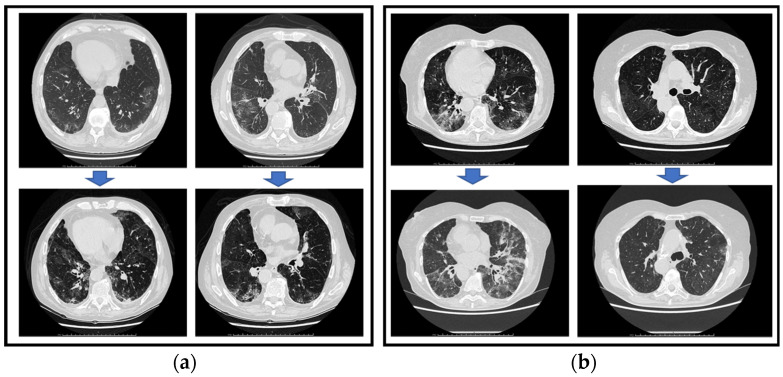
Chest CT scan before (upper row) and after (lower row) CP treatment. (a) Case 1; (b) Case 2.
